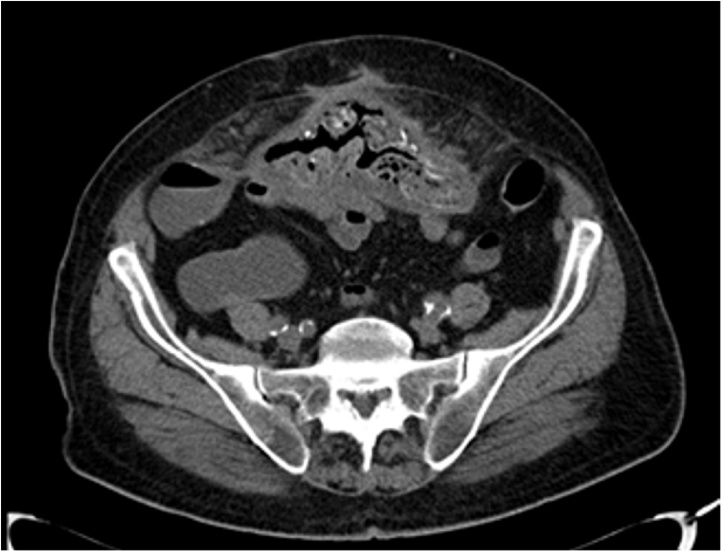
Preoperative CT scan demonstrating small bowel loops adherent to the mesh.
